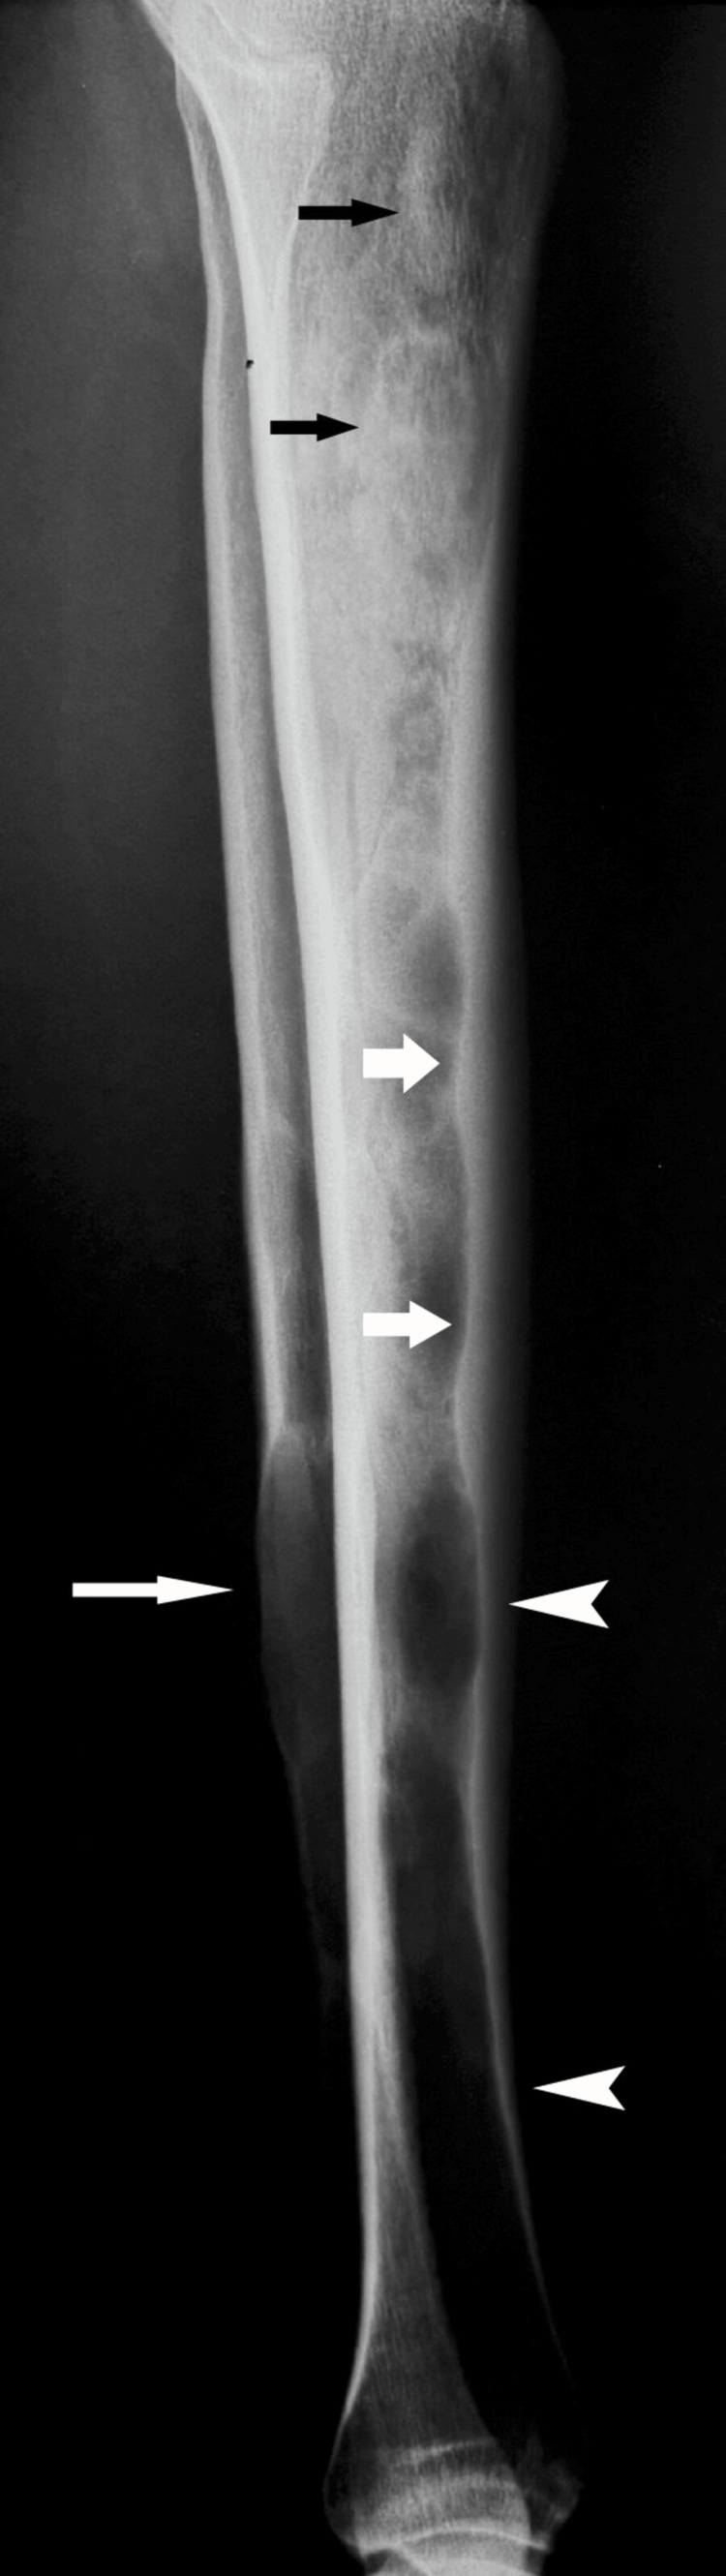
Lateral radiograph of the lower limb demonstrates multiple diaphyseal radiodense (arrows) and radiolucent lesions (arrowheads) in the tibia and fibula. Cortical scalloping (thick arrows) is seen. Note the large, lytic expansile lesion (long arrow) within the diaphysis of the distal fibula.
